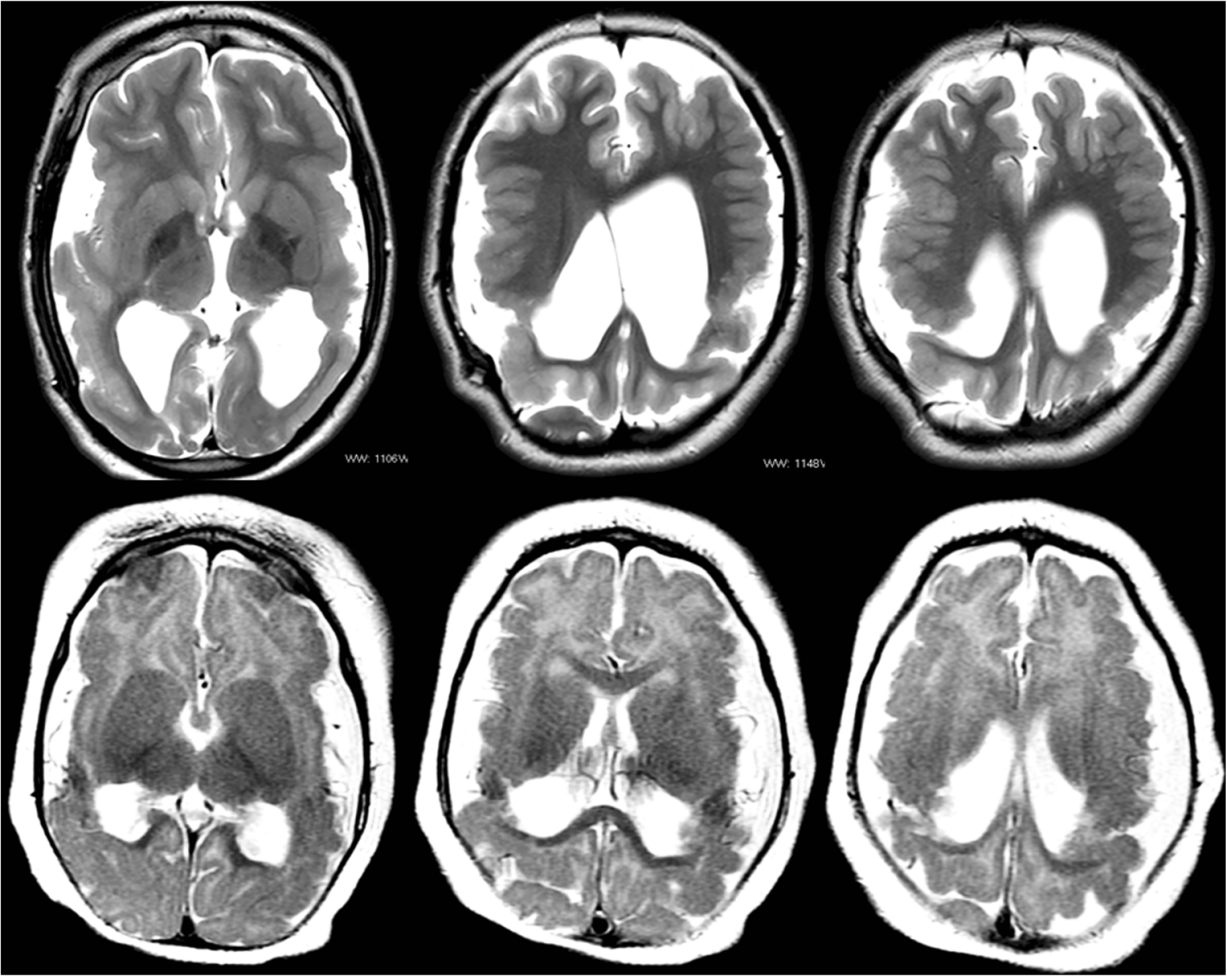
MR images of a 7-year-old child (upper pane of the images) with bilateral schizencephaly (type 2) and known EMX2 genetic mutation and MR images of a 7-month-old child with a strong family history of schizencephaly but no genetic testing at the time of the MR study (lower pane of the images). The two cases show similar imaging features as discussed in the text
